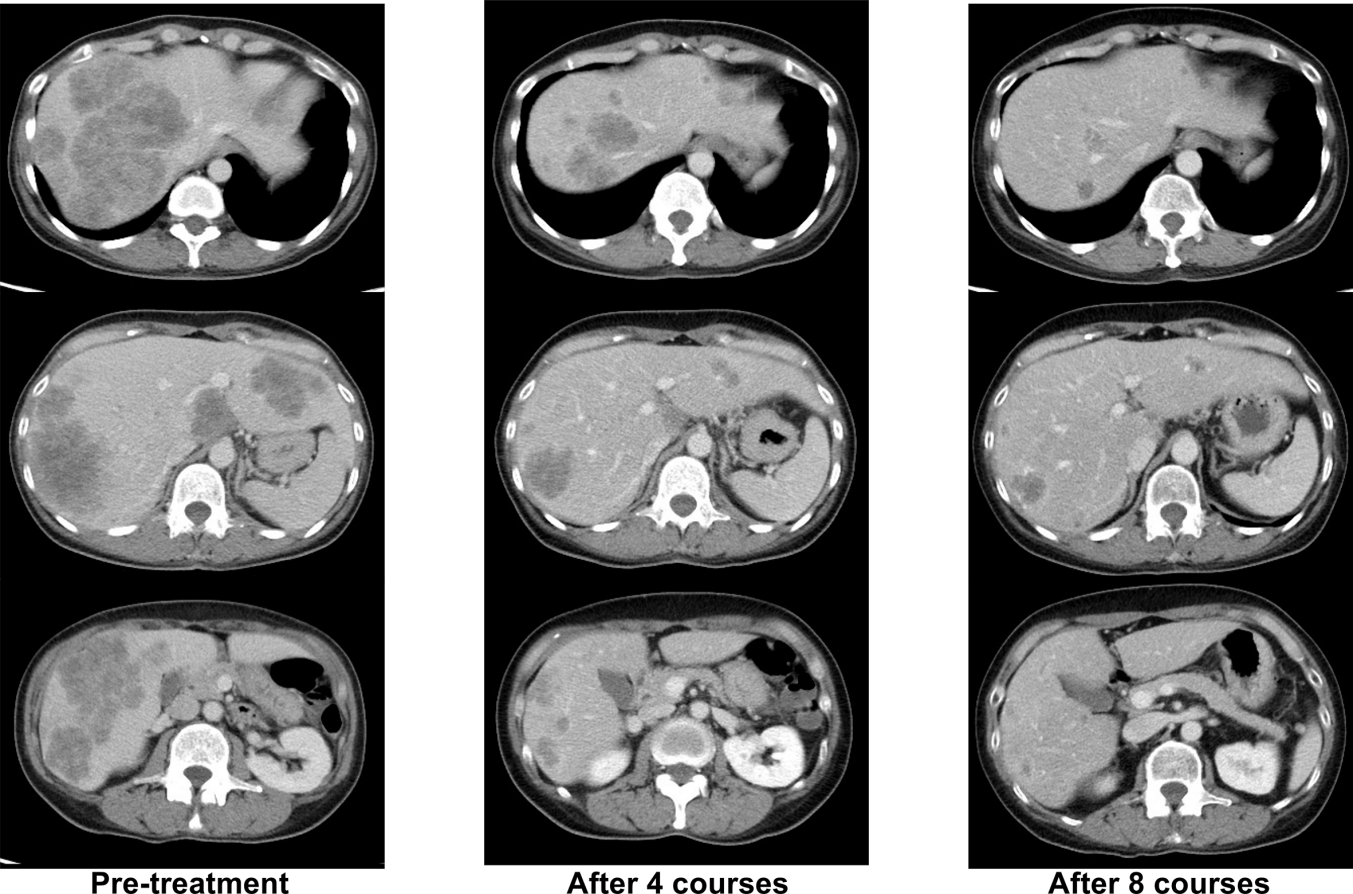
Representative computed tomography (CT) images of liver lesions during ^-^firstline mFOLFOX6+cetuximab treatment.
